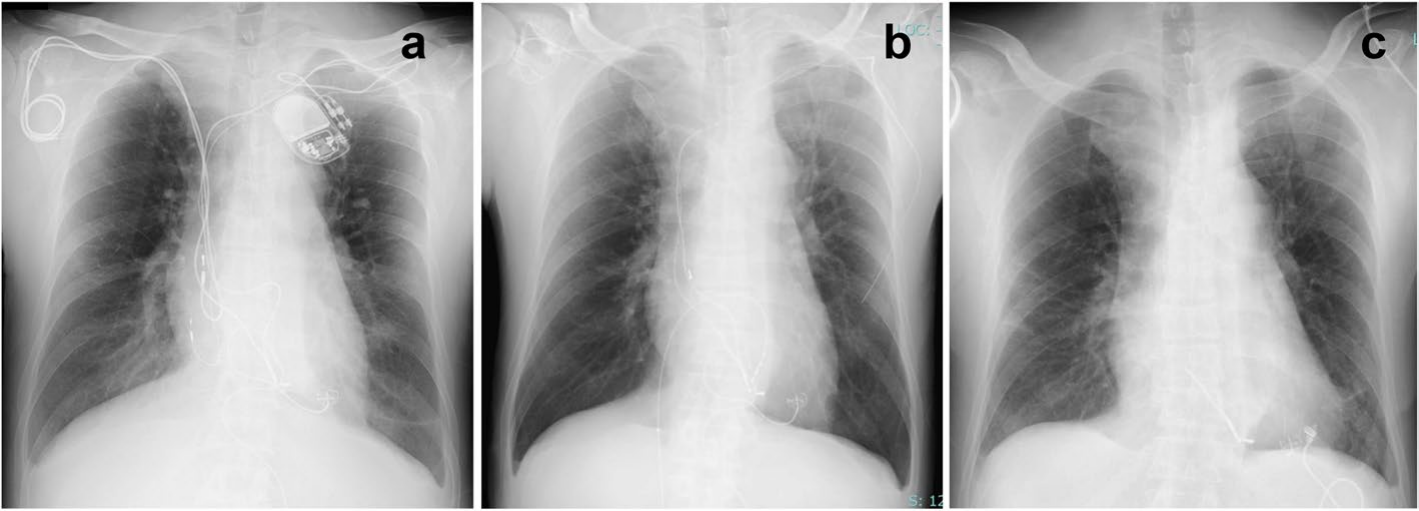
Perioperative chest radiography. A toral of four leads were placed transvenously and an epicardial lead was left in the diaphragmatic plane (a). Two RV leads was remained after transvenous lead extraction (b). Only a part of the RV lead remained and a new epicardial lead was placed (c)
